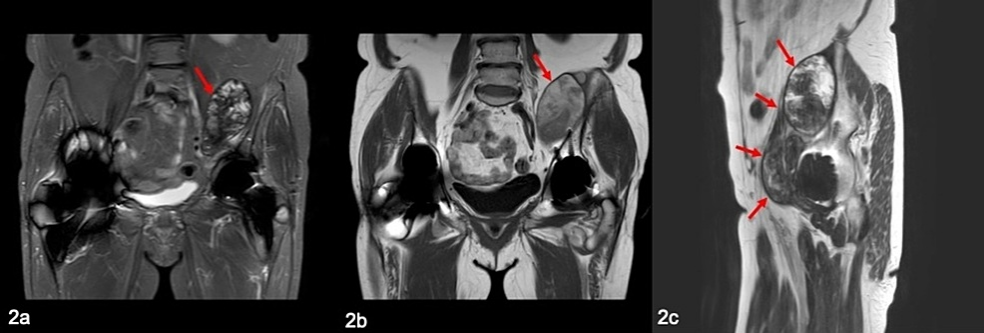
MRI of enlarging pseudotumour (red arrows) with evidence of haemorrhage in 2018. (a) Coronal STIR; (b) coronal T1; (c) sagittal T2. MRI: magnetic resonance imaging; STIR: short TI inversion recovery
